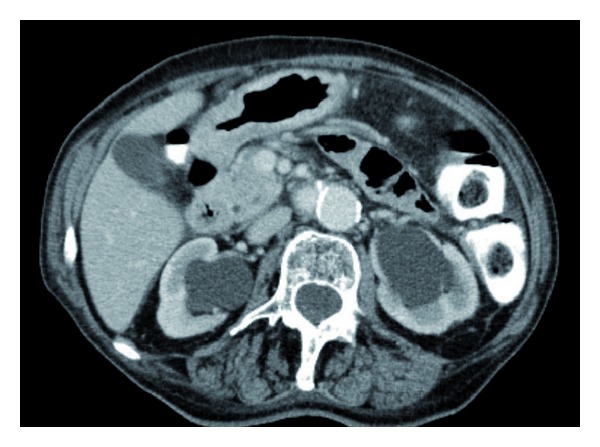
Axial computed tomography scan of the abdomen showing bilateral hydronephrosis without enlarged retroperitoneal lymph nodes.
